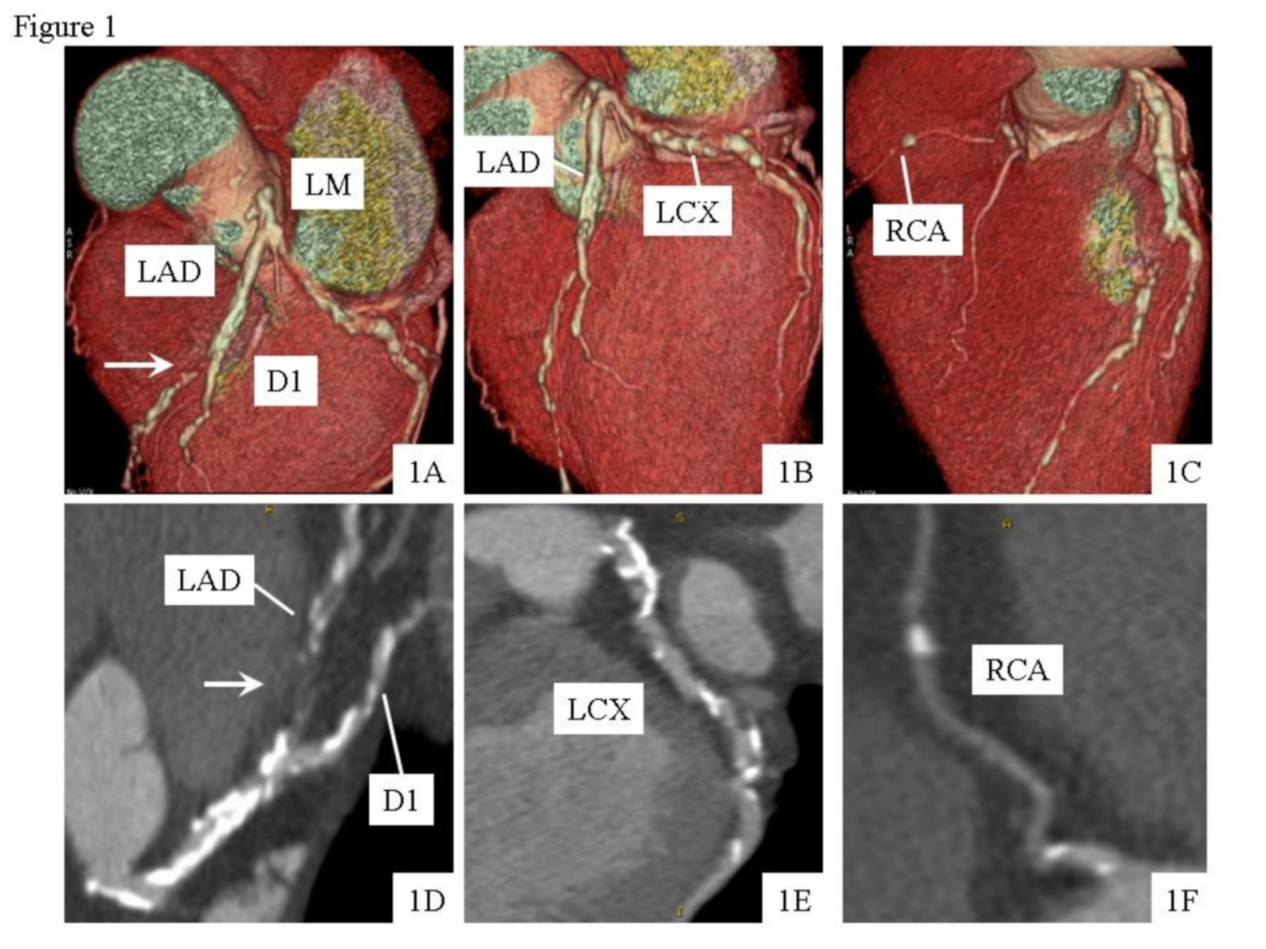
MDCT volume rendering (upper panels 1A, 1B, 1C) and multiplanar reconstruction (lower panels 1D, 1E, 1F) showing the small coronary size and large coronary calcifications of the epicardial coronary vessels. The white arrow indicate an occlusion of the middle portion of LAD (1A, 1D). *D1 = first diagonal branch; LAD = left anterior descending coronary artery; LCX = left circumflex artery; LM = left main artery; MDCT = multidetector computed tomography; RCA = right coronary artery.*
